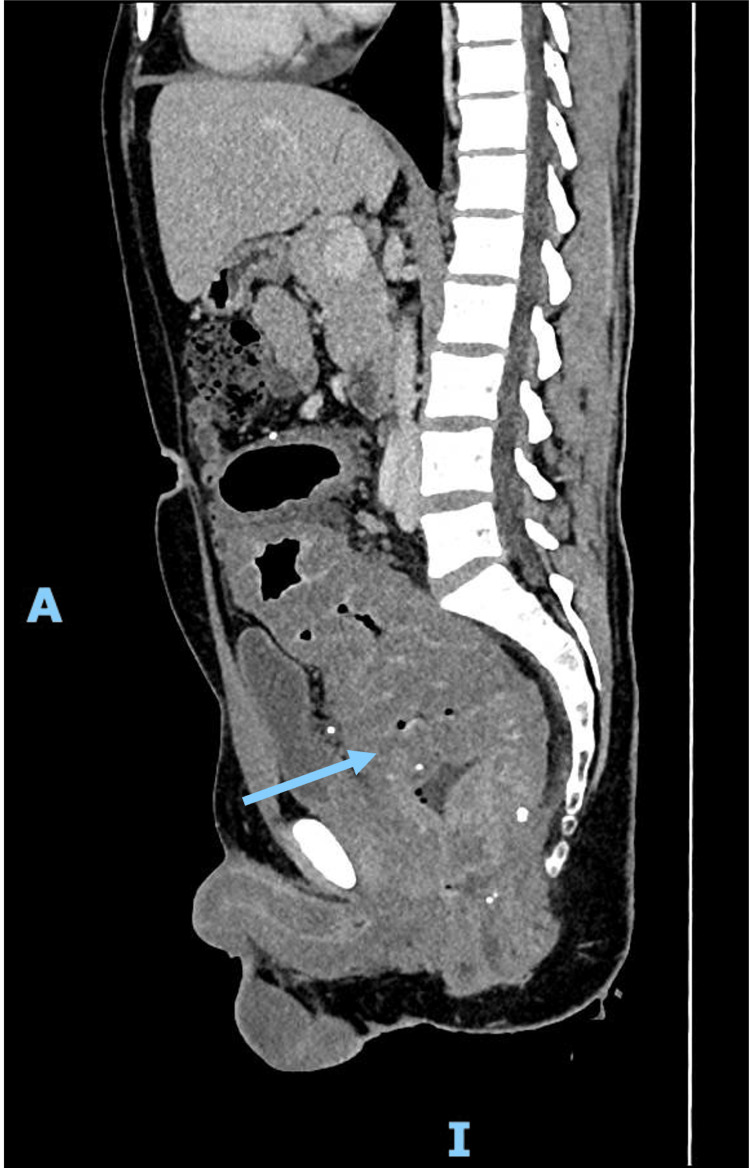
CT scan of the abdomen and pelvis Marked diffuse parietal thickening of the anal canal, rectum, and sigmoid colon, extending up to the transition with the left colon over approximately 50 cm. The findings are more prominent in the rectum and anal canal, where there is apparent luminal narrowing.
